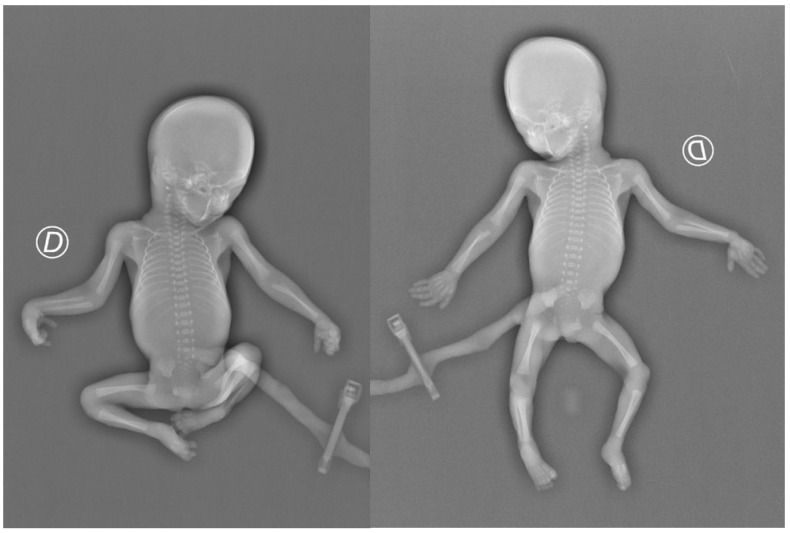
Repetition of X-ray after extension of the limbs to limit artefacts.
